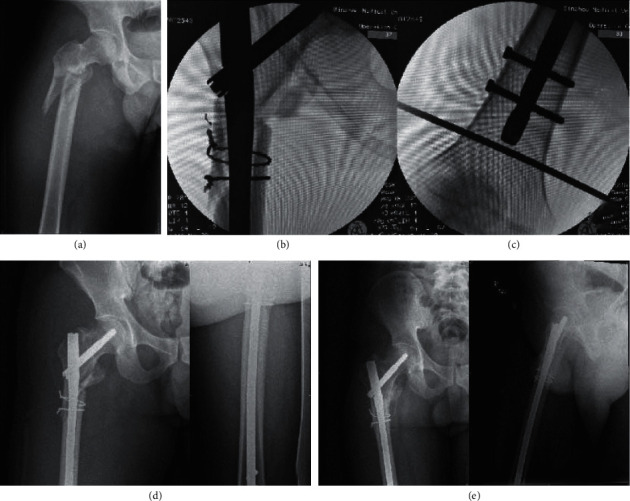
Preoperative X-ray of the patient, Seinsheime classification III a (a). During the operation, the length of the fracture was restored by traction with the retractor. Then, the broken end of the fracture was cut open, and the fracture was anatomically reduced, fixed with two wires, and fixed with a full-length intramedullary nail (b). X-ray examination was performed 2 days after surgery. It showed that the fracture had a good reduction, and there was no valgus deformity (c). Three months after the operation, the fracture line disappeared and healed well (d).
